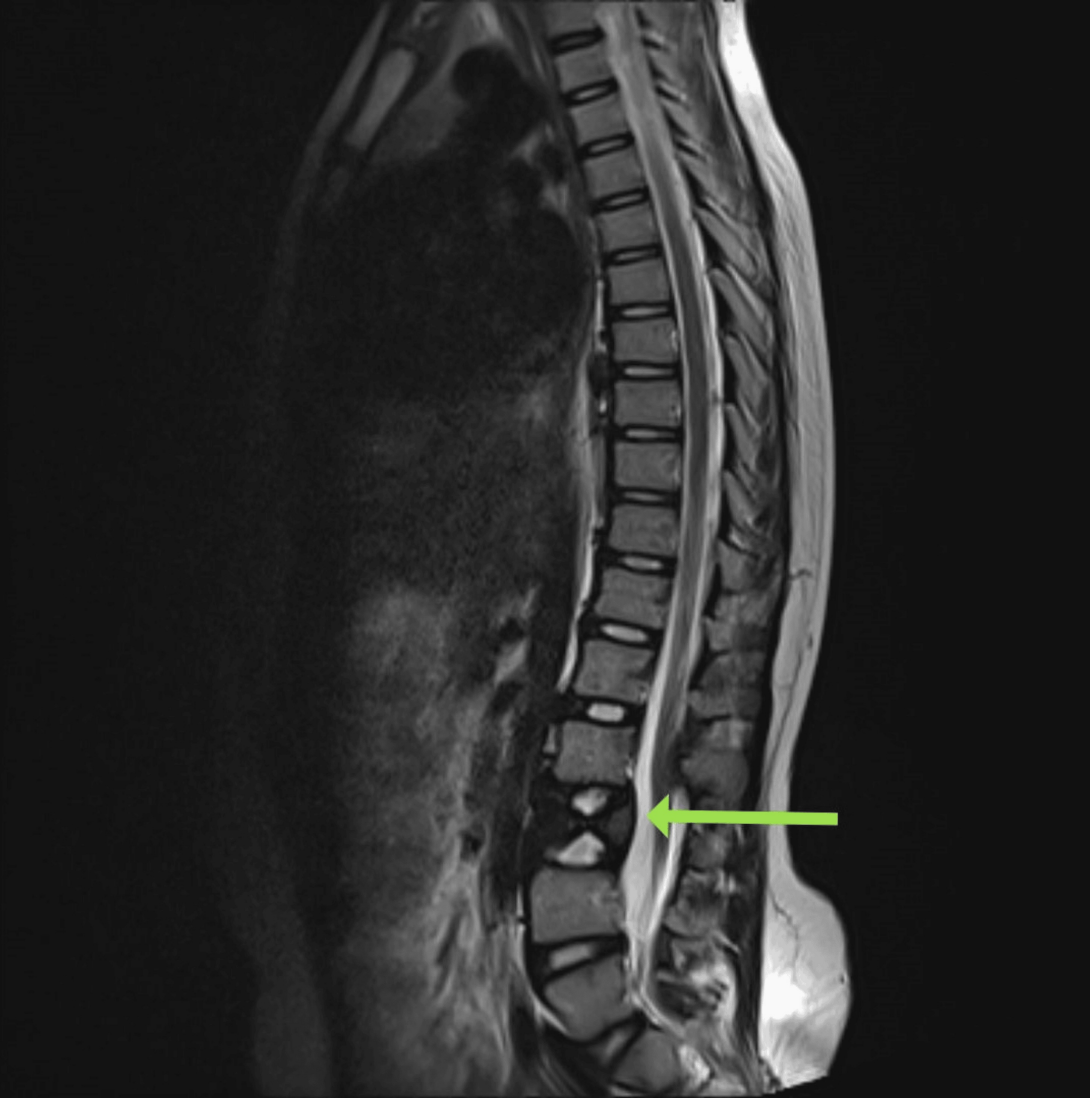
MRI image The arrow shows the L2 hemivertebra in the sagittal section. At the level of the L1 vertebra, the cord appears adherent to the posterior dura-tethered cord.
